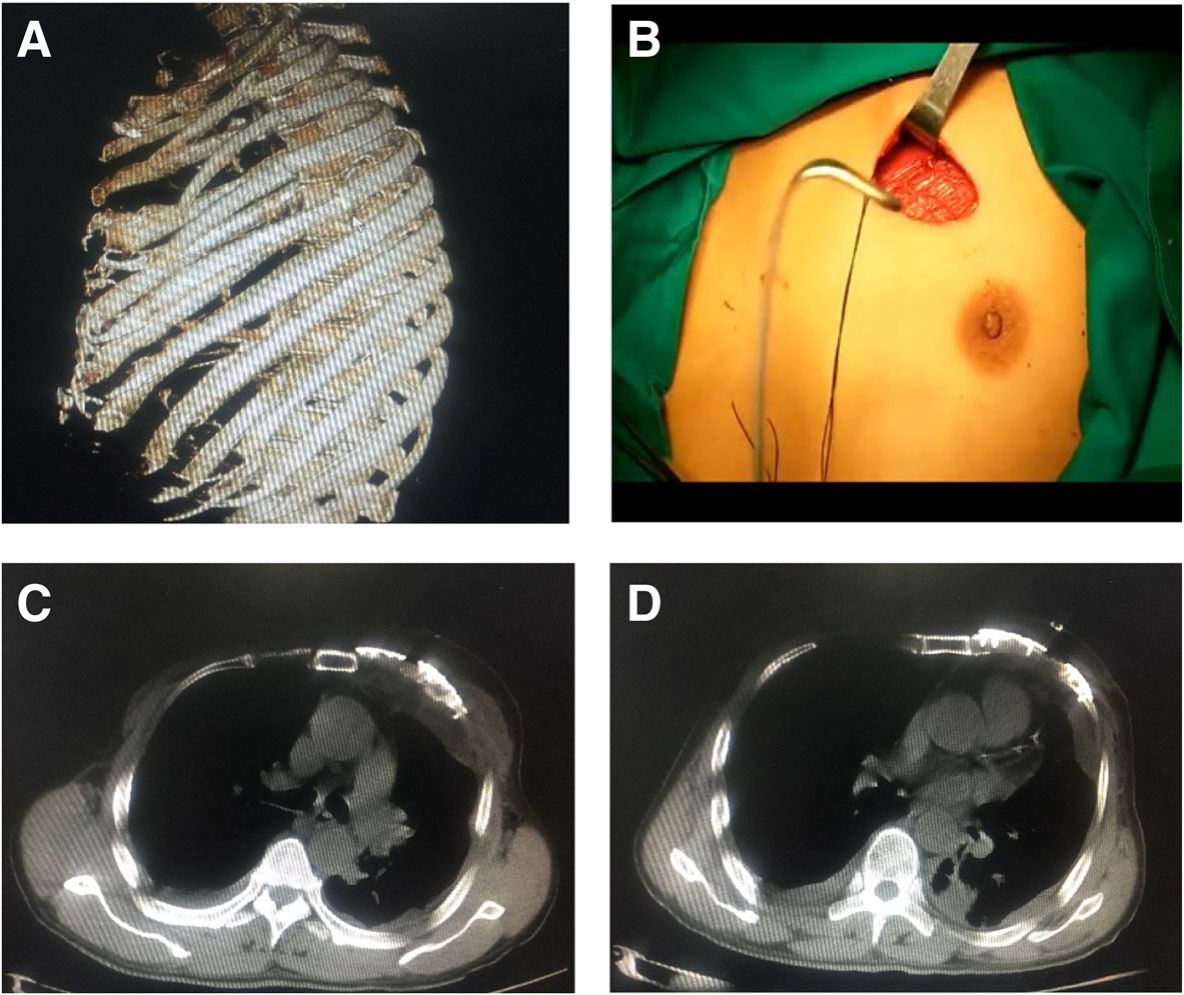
No.4 patient (a) Preoperative scanning revealed 3–6 anterior multiple fractures in the left side involving the cartilage. b During the surgery, a 6-cm chest incision was made. c, d The postoperative CT locking plate position and thoracic morphology were satisfactory
